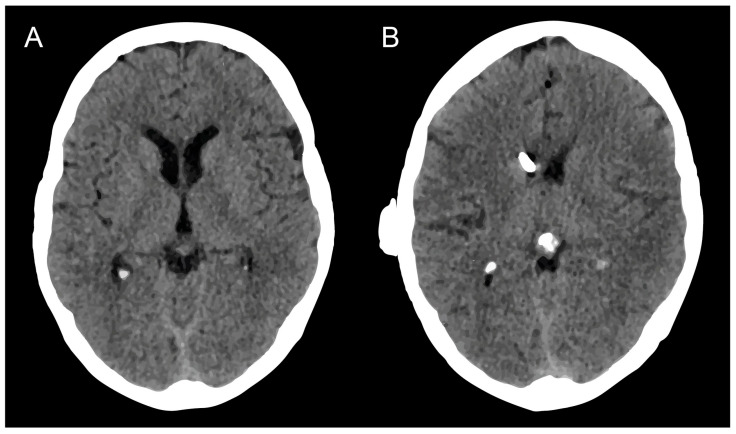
(A) Cranial CT scan on the day before surgery and (B) cranial CT scan on the postoperative day, showing a reduction in ventricular size in a patient with occlusive HC due to LC. The end of the cranial shunt catheter is visible projecting onto the foramen of Monroi in image (B). HC, hydrocephalus; LC, leptomeningeal carcinomatosis.
